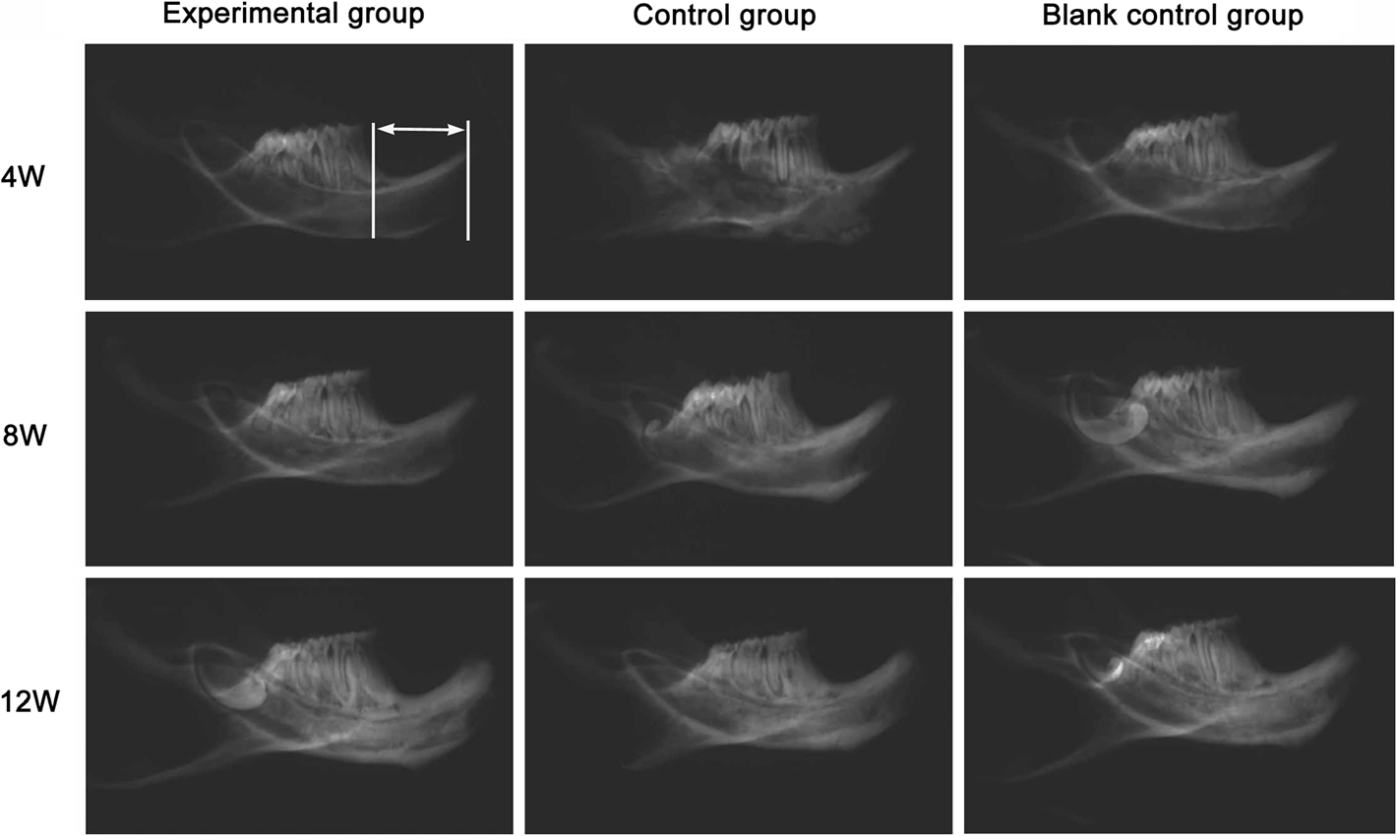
Soft X-ray images of mandibles at each predetermined time. The morphometric measurement on residual alveolar ridge by radiation is focused on the distance between apex of mandible ridge of incisor and the highest point of the mesial alveolar bone of first molar, just as the arrow showed.
